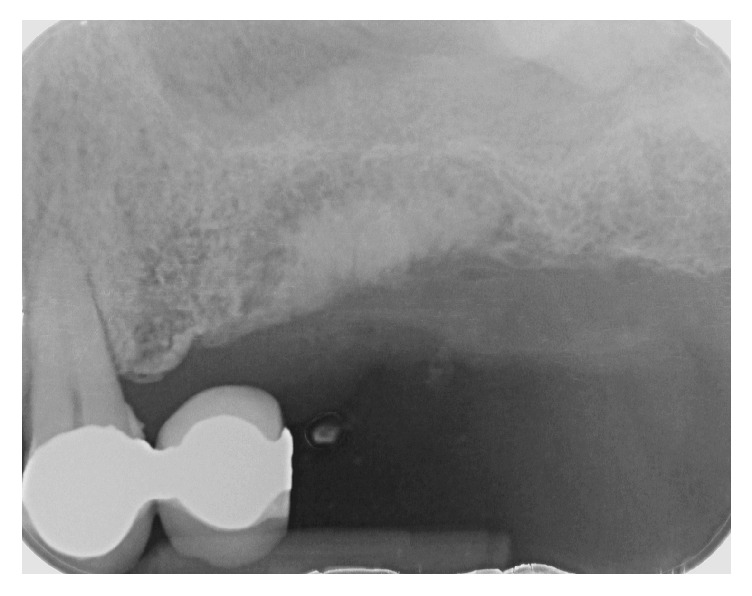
Intraoral radiograph collected two months later showing the limited thickness of the residual posterior ridge.
